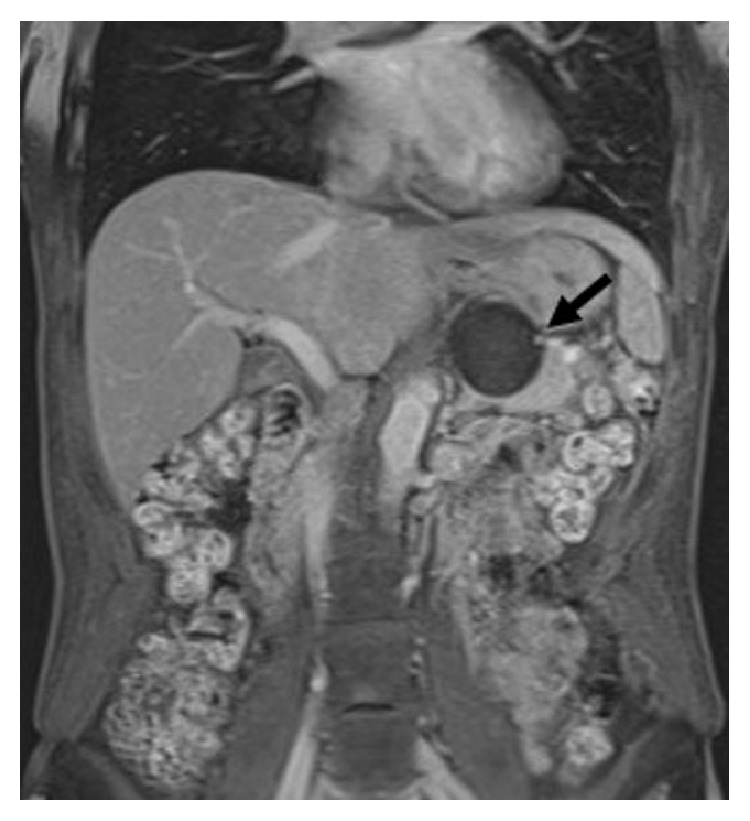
Axial T1-weighted magnetic resonance imaging showing homogeneous low signal intensity of the adrenal cystic lesion. A mural nodule with contrast enhancement (arrow) is observed.
